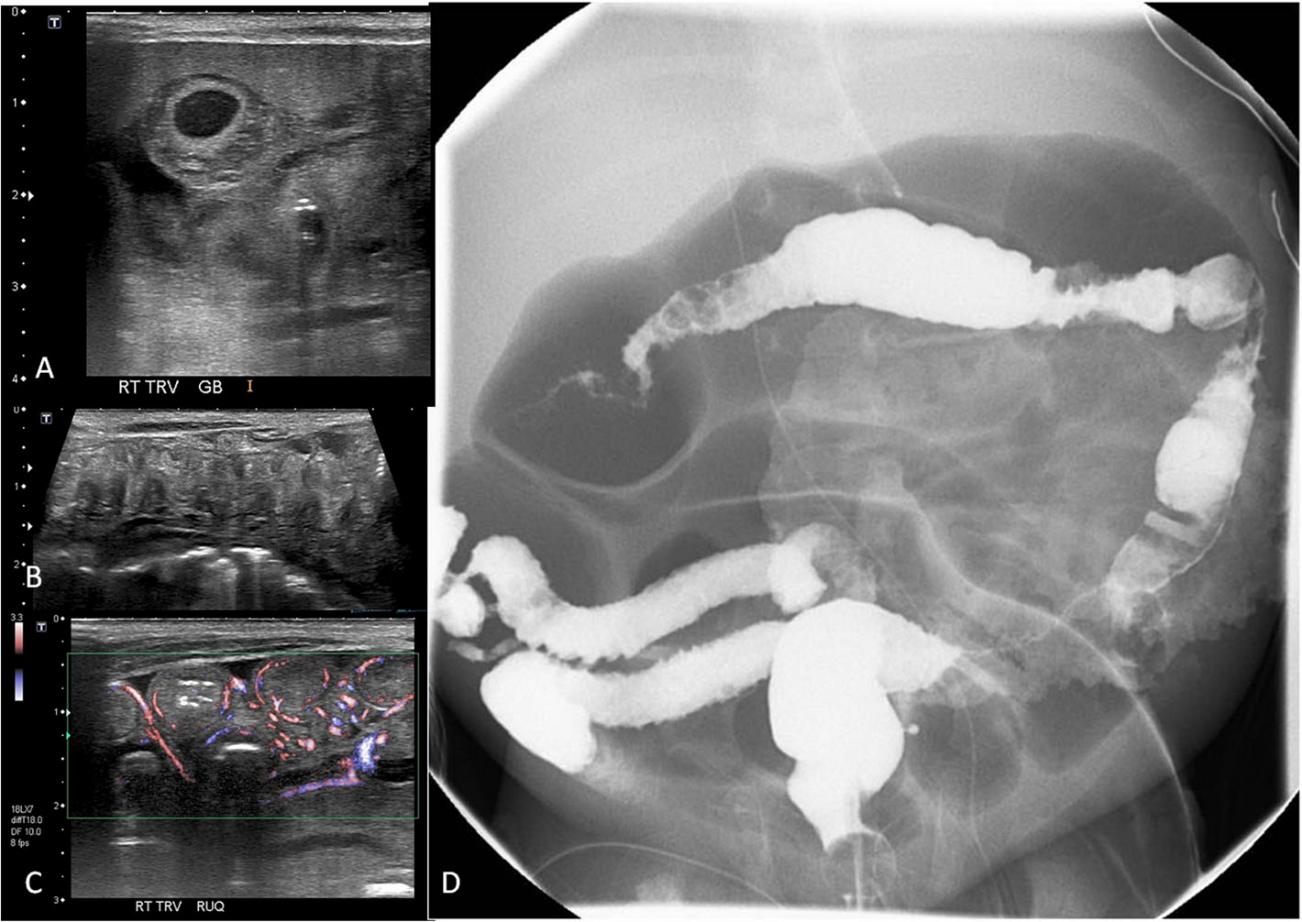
A 5-week-old baby girl with bilious vomiting and abdominal distension. a–c Selected images of abdominal ultrasound demonstrate a marked gallbladder wall thickening and (b) small bowel wall thickening and hyperemia and (c) transverse colon wall thickening. There was lack of peristalsis on real time ultrasound and moderate ascites (not shown). There was no pneumatosis intestinalis. d Frontal fluoroscopic view of contrast enema performed at 8 weeks of life due to persistent abdominal distension and bloody diarrhea: the contrast is filling the colon, terminal ileum, and appendix: there is mildly reduced caliber of the colon with multiple areas of wall irregularities of the colon and terminal ileum. Note the massively dilated small bowel loops at the center of the abdomen. This patient is found to have IL-10 deficiency and positive genetic testing (IL10RB)
